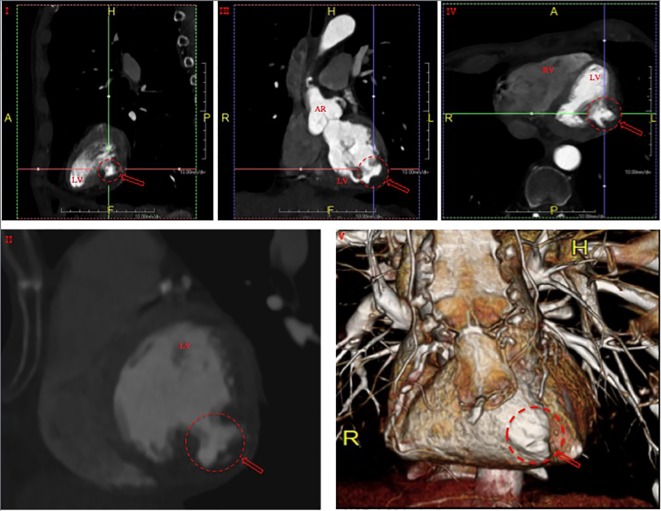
Cardiac computed tomography demonstrating a 1 × 2 cm outpouching along the inferolateral left ventricular wall at the mid cavity level in sagittal (I, II), coronal (III), axial (IV) and three-dimensionally reconstructed (V) views, consistent with pseudoaneurysm (arrows). RV: right ventricle; LV: left ventricle; AR: aortic root.
